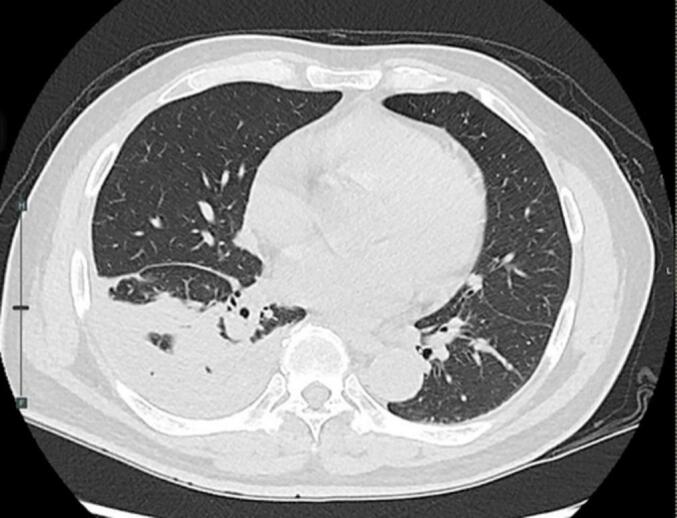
CT scan showing retained haemothorax and passive atelectasis of the lung parenchyma.
